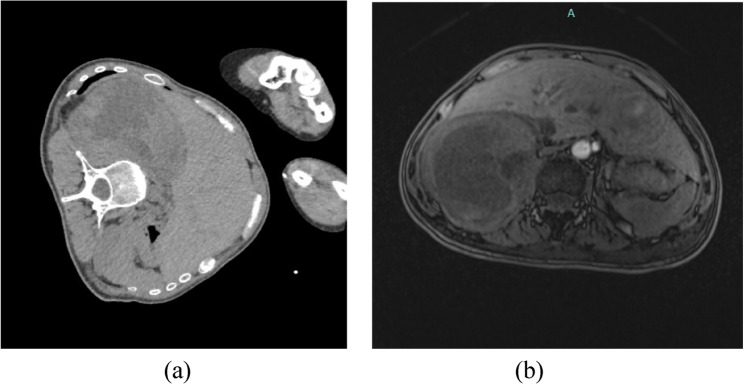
Imaging of the pheochromocytoma. a a mass at the upper pole of the right kidney on preoperative CT. b a 10 × 10 cm right adrenal mass on MRI
